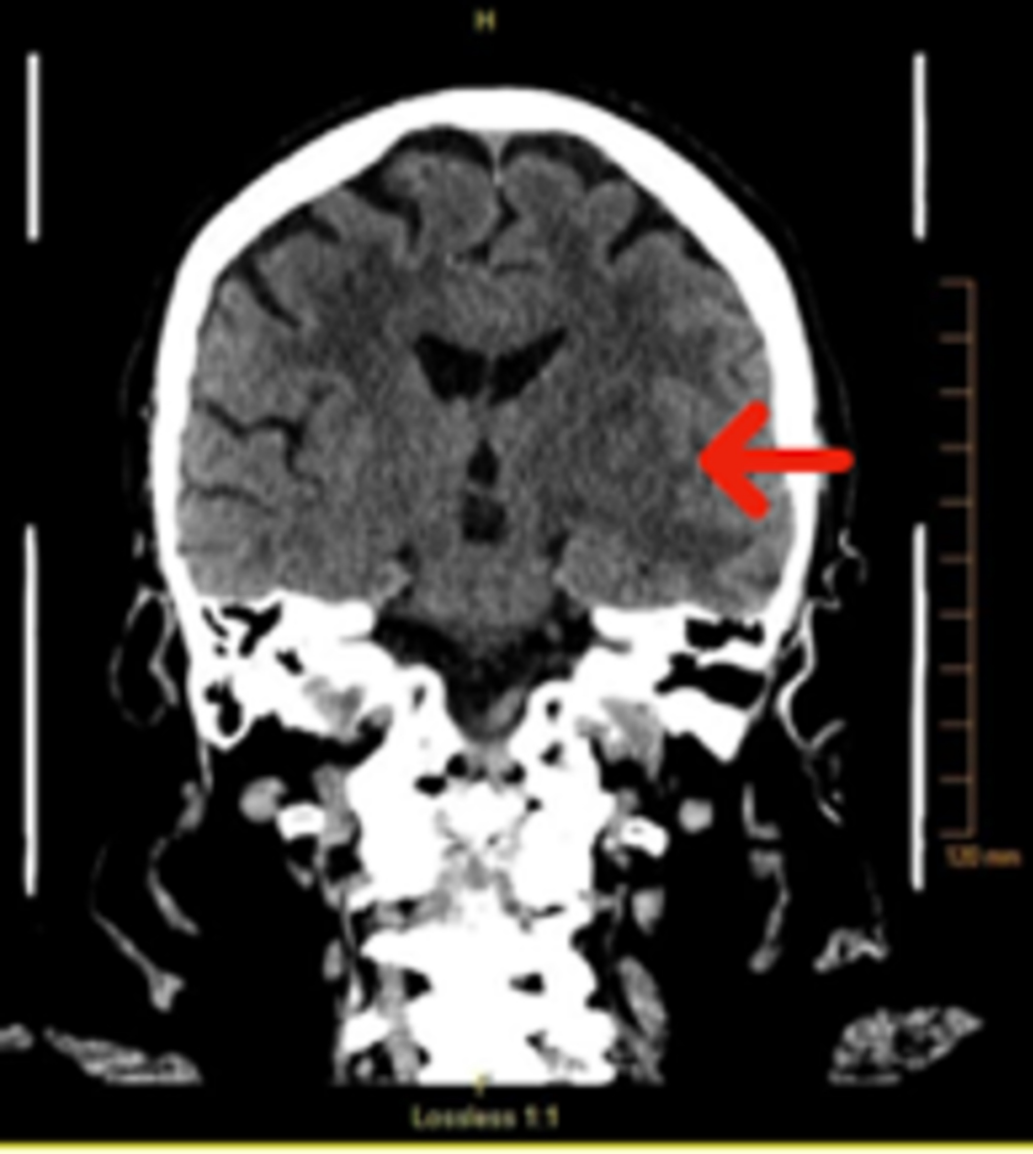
CT of the head without contrast The image showed evidence of bilateral edema and lesions throughout the basal ganglia (left greater than the right, red arrow). There was also mild mass effect on the left lateral ventricle CT: computed tomography
